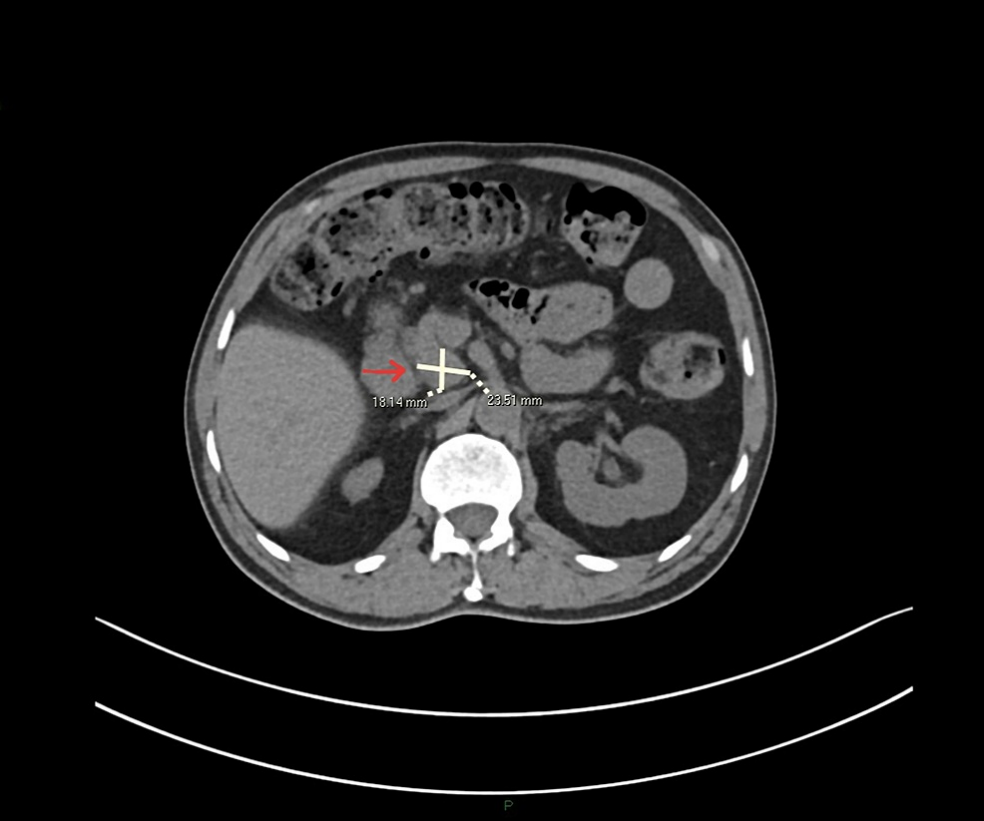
CT abdomen. Peri pancreatic lymph nodes (arrow) on initial CT scan performed during pre-transplant workup.
